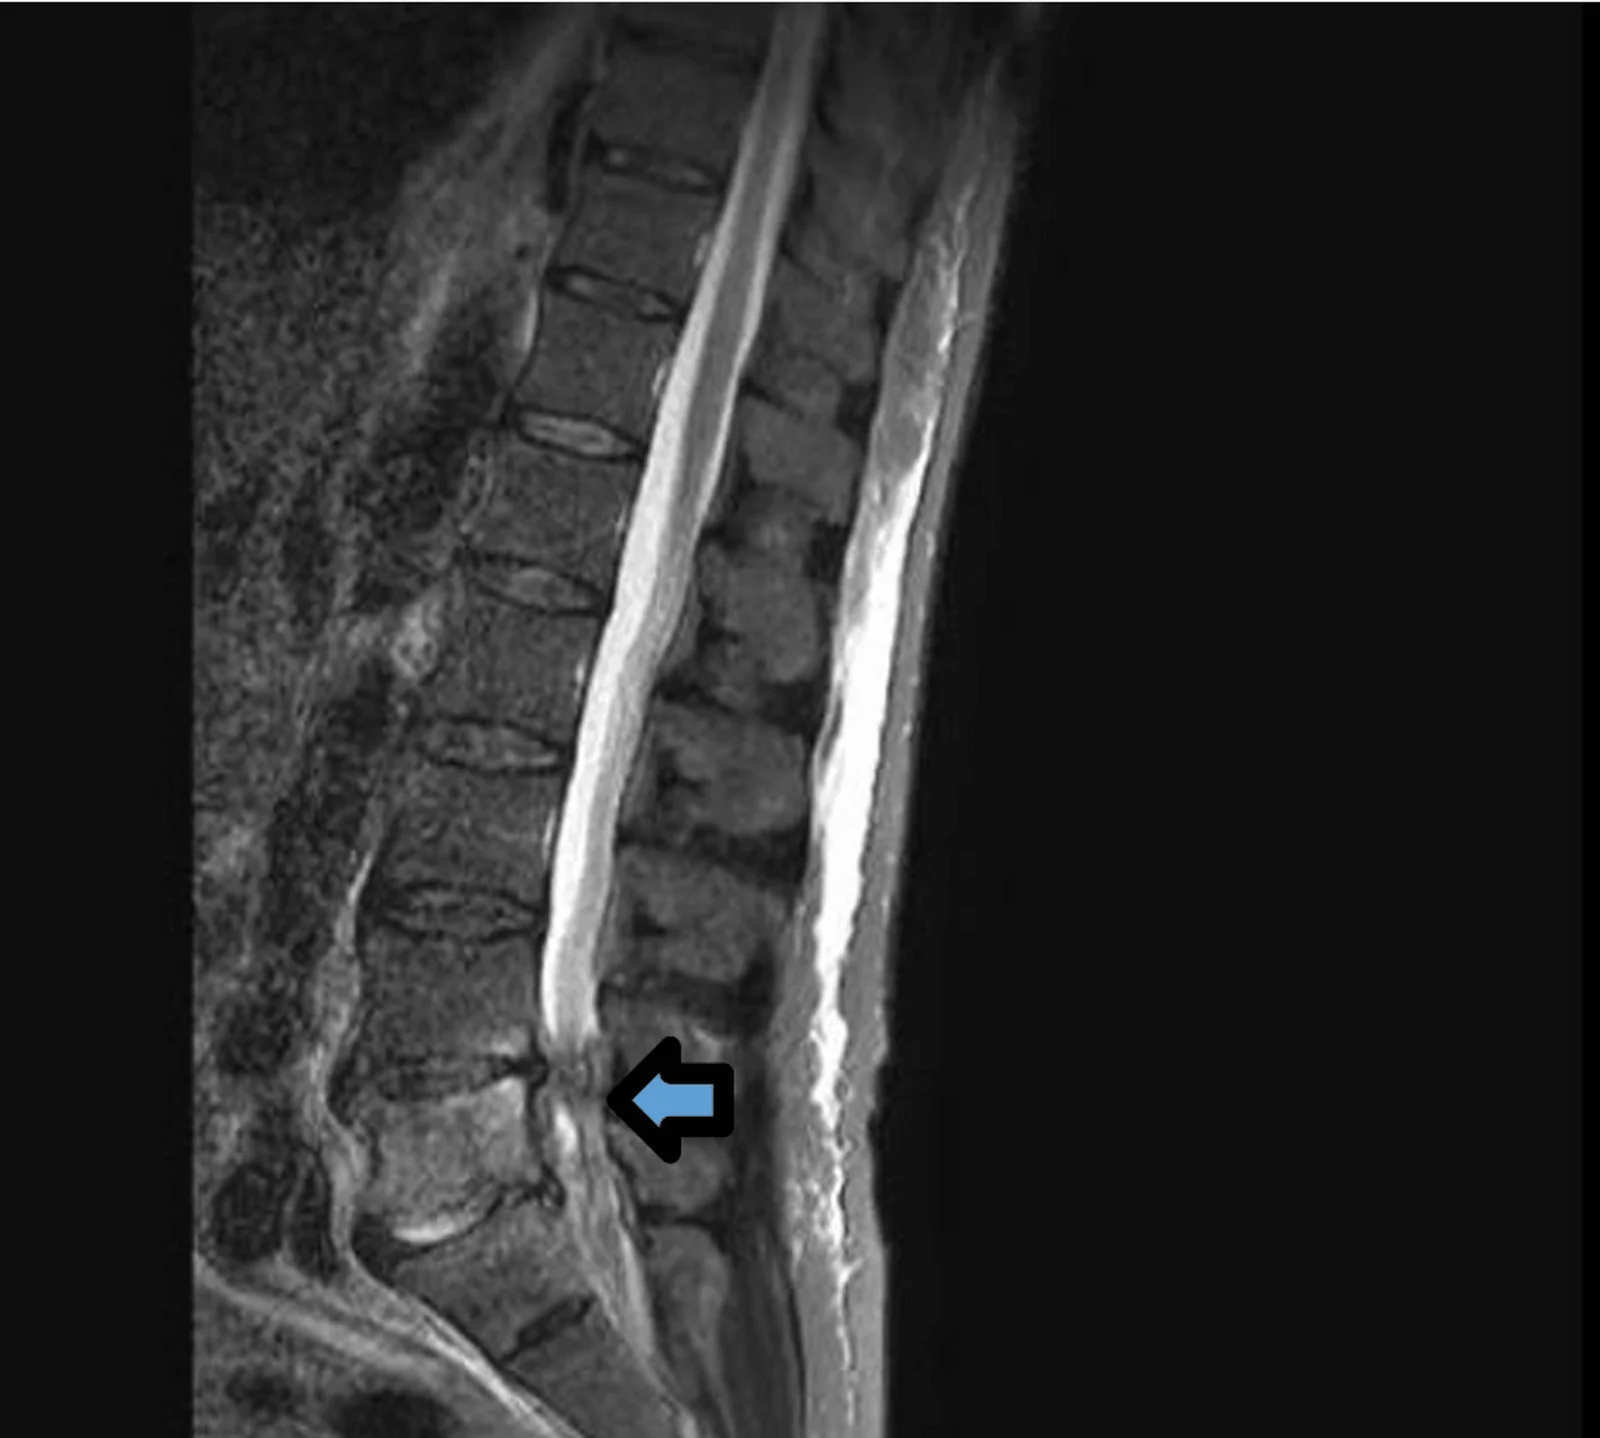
Initial whole-spine MRI showing multilevel degenerative cervical and lumbar spondylotic changes without evidence of discitis, vertebral osteomyelitis, or epidural abscess (blue arrow).
